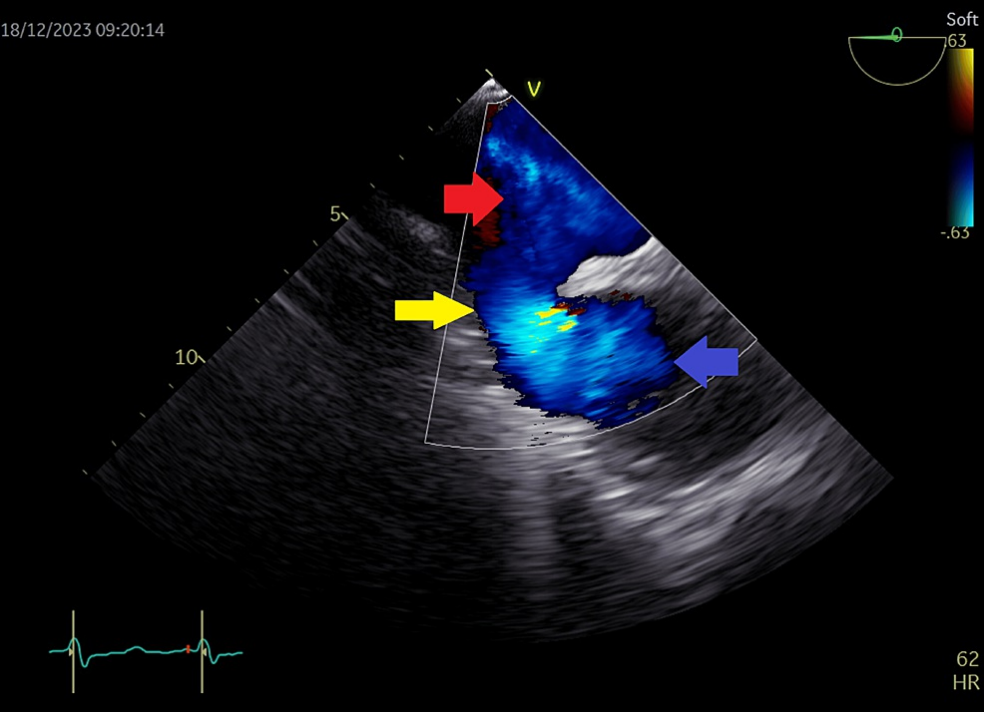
Transesophageal echocardiogram at the level of left atrium and right atrium showing color flow across the sinus venosus atrial septal defect with left to right shunt (yellow arrow). The red arrow shows flow from the left atrium to the right atrium (blue arrow).
